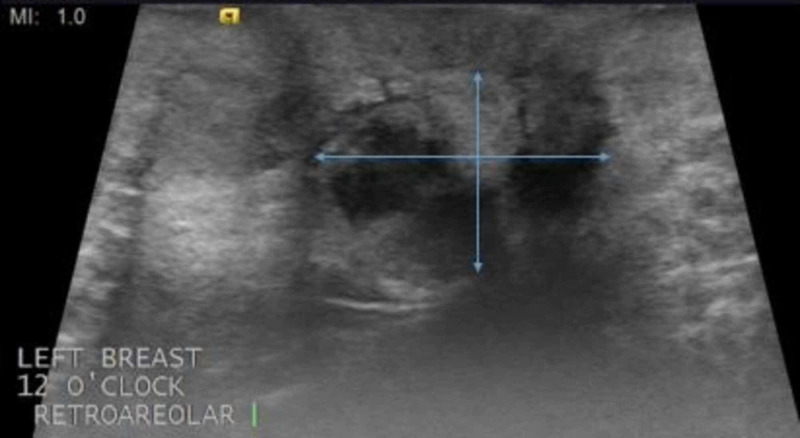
Ultrasound of the left breast in the longitudinal plane Ultrasound performed in the longitudinal plane again shows the mass; a biopsy is recommended for further evaluation. Borders of the mass are shown by the blue arrows
